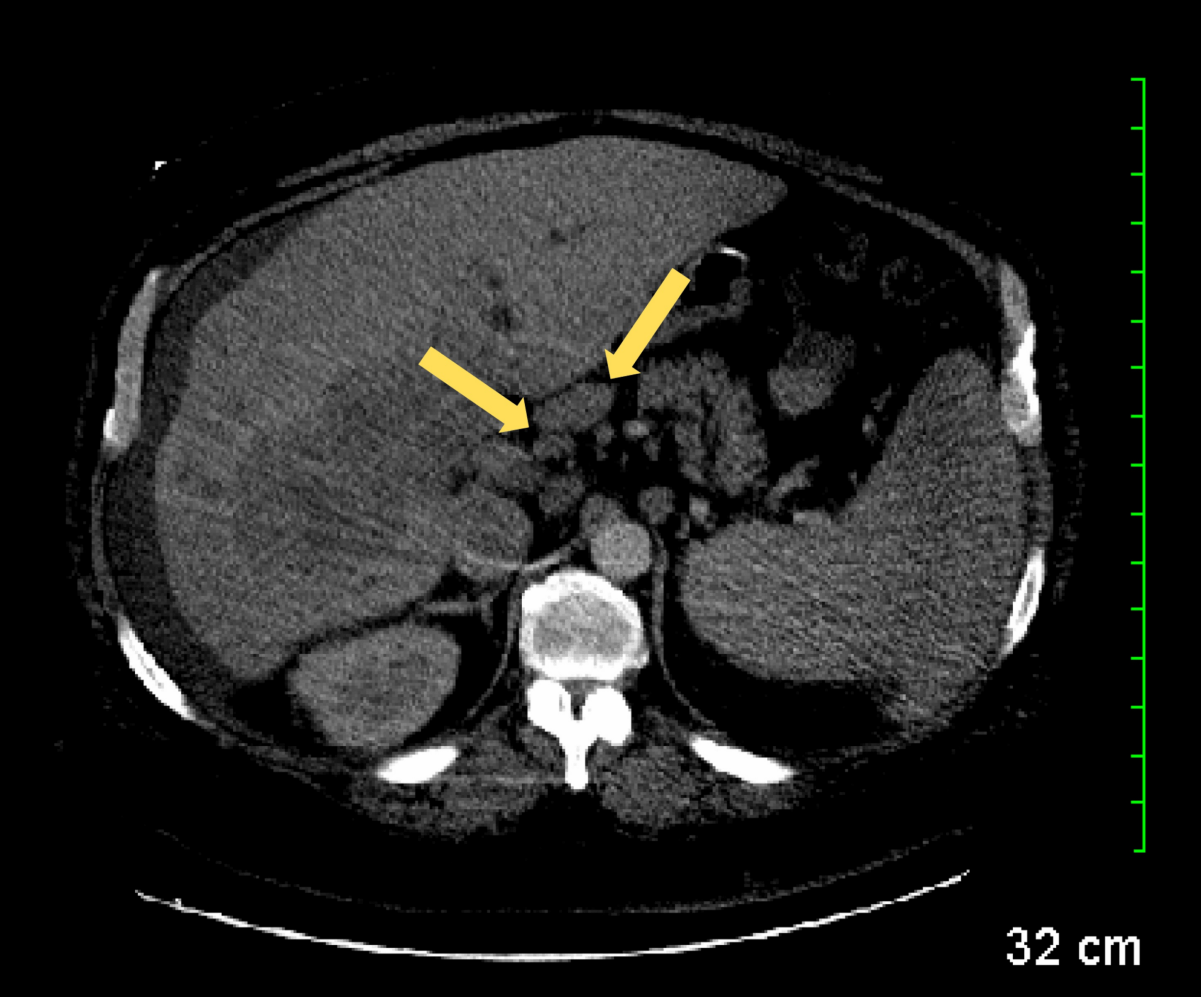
CT scan revealing prominent lymph nodes (yellow arrows) in the porta hepatis region. CT: computed tomography
